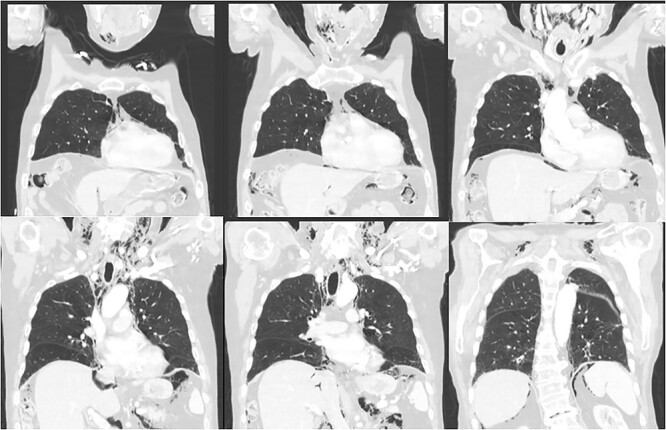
CT scan of the chest demonstrating subcutaneous emphysema and extensive free gas in the mediastinum and retroperitoneum.
